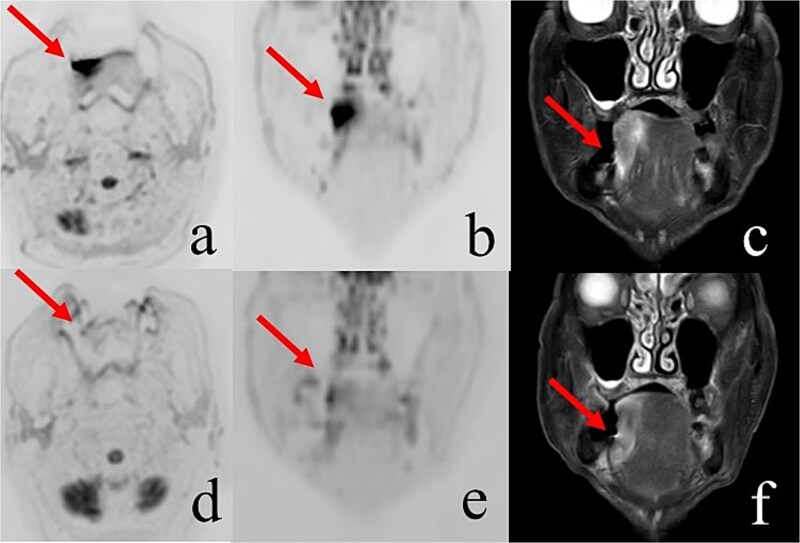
Magnetic resonance imaging findings before and after treatment*.* The images in the top row (a, b, and c) are from before the Quad Shot, and the images in the bottom row (d, e, and f) are from 2 months after the Quad Shot. Diffusion-weighted images show abnormal signals before treatment (a and b); however, these signals disappeared 2 months after treatment (d and e). No changes are visible in the short tau inversion recovery (STIR) images (c and f), as diffusion-weighted imaging reflects changes in the internal structure of the lesion earlier than STIR.
